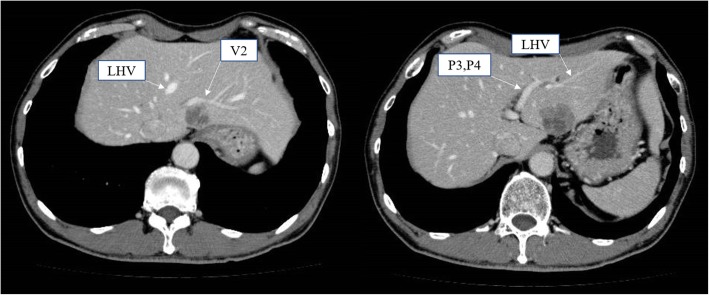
Abdominal contrast-enhanced CT revealed a hypovascular tumor in the left lateral section. V2, drainage vein of the lateral superior section; LHV, left hepatic vein; P3, lateral inferior branch of the portal vein; P4, left paramedian branch of the portal vein
